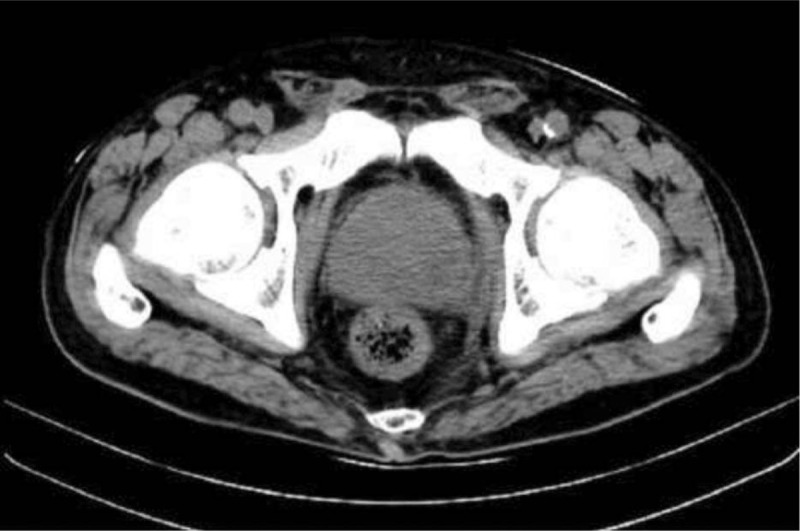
Pelvic CT showed almost complete resolution of the EPA 1 month after discharge.
